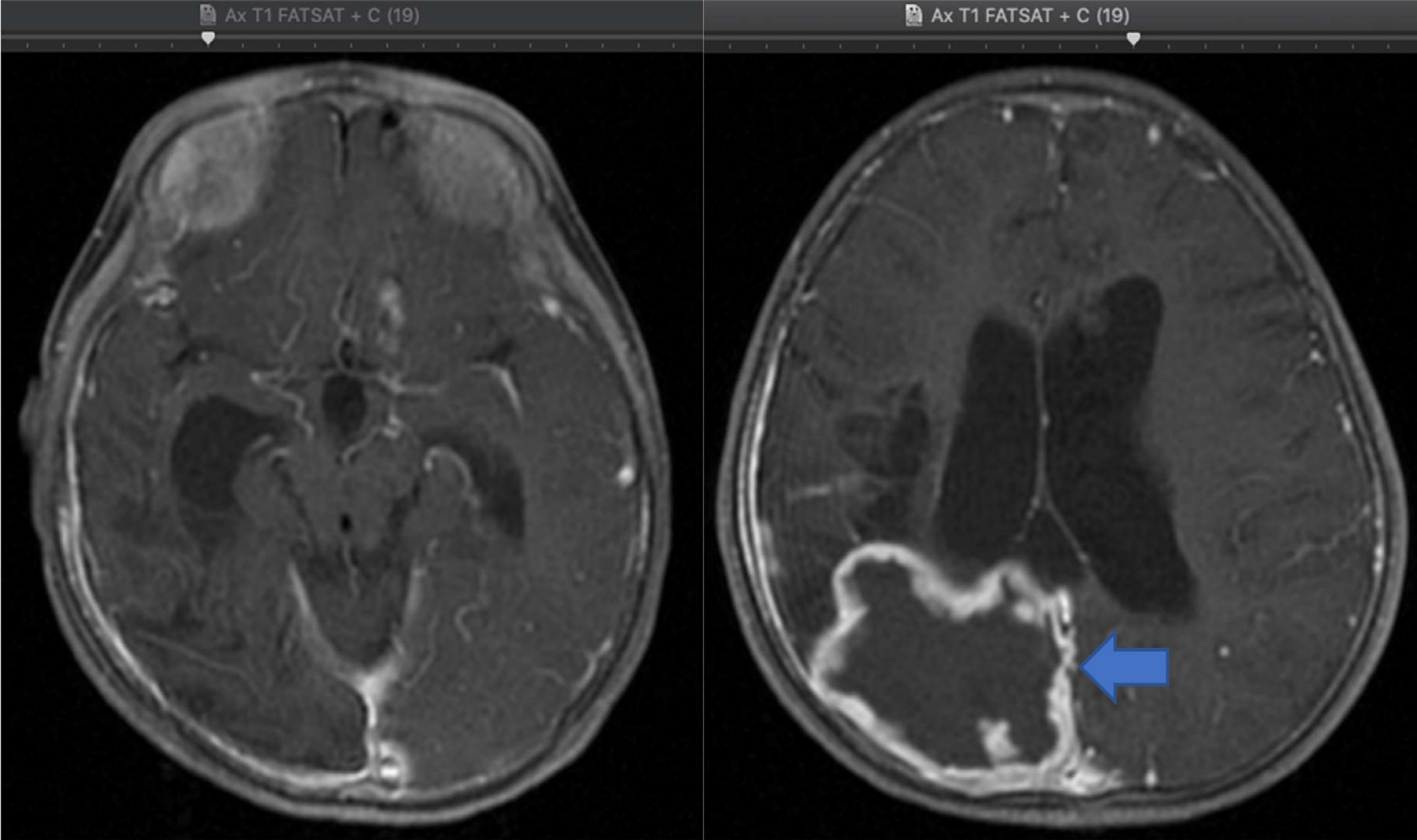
The axial section of the T1 Fatsat with contrast MRI, On intravenous gadolinium contrast administration, pachymeningeal enhancement in the right temporo-parieto-occipital region can be seen, with the enhancement in the left cisterna basalis and rim contrast enhancement in the cortical-subcortical lesion of the right occipital lobe which may represent meningoencephalitis with infected cystic lesion, strongly suggesting tuberculoma (blue arrow).
